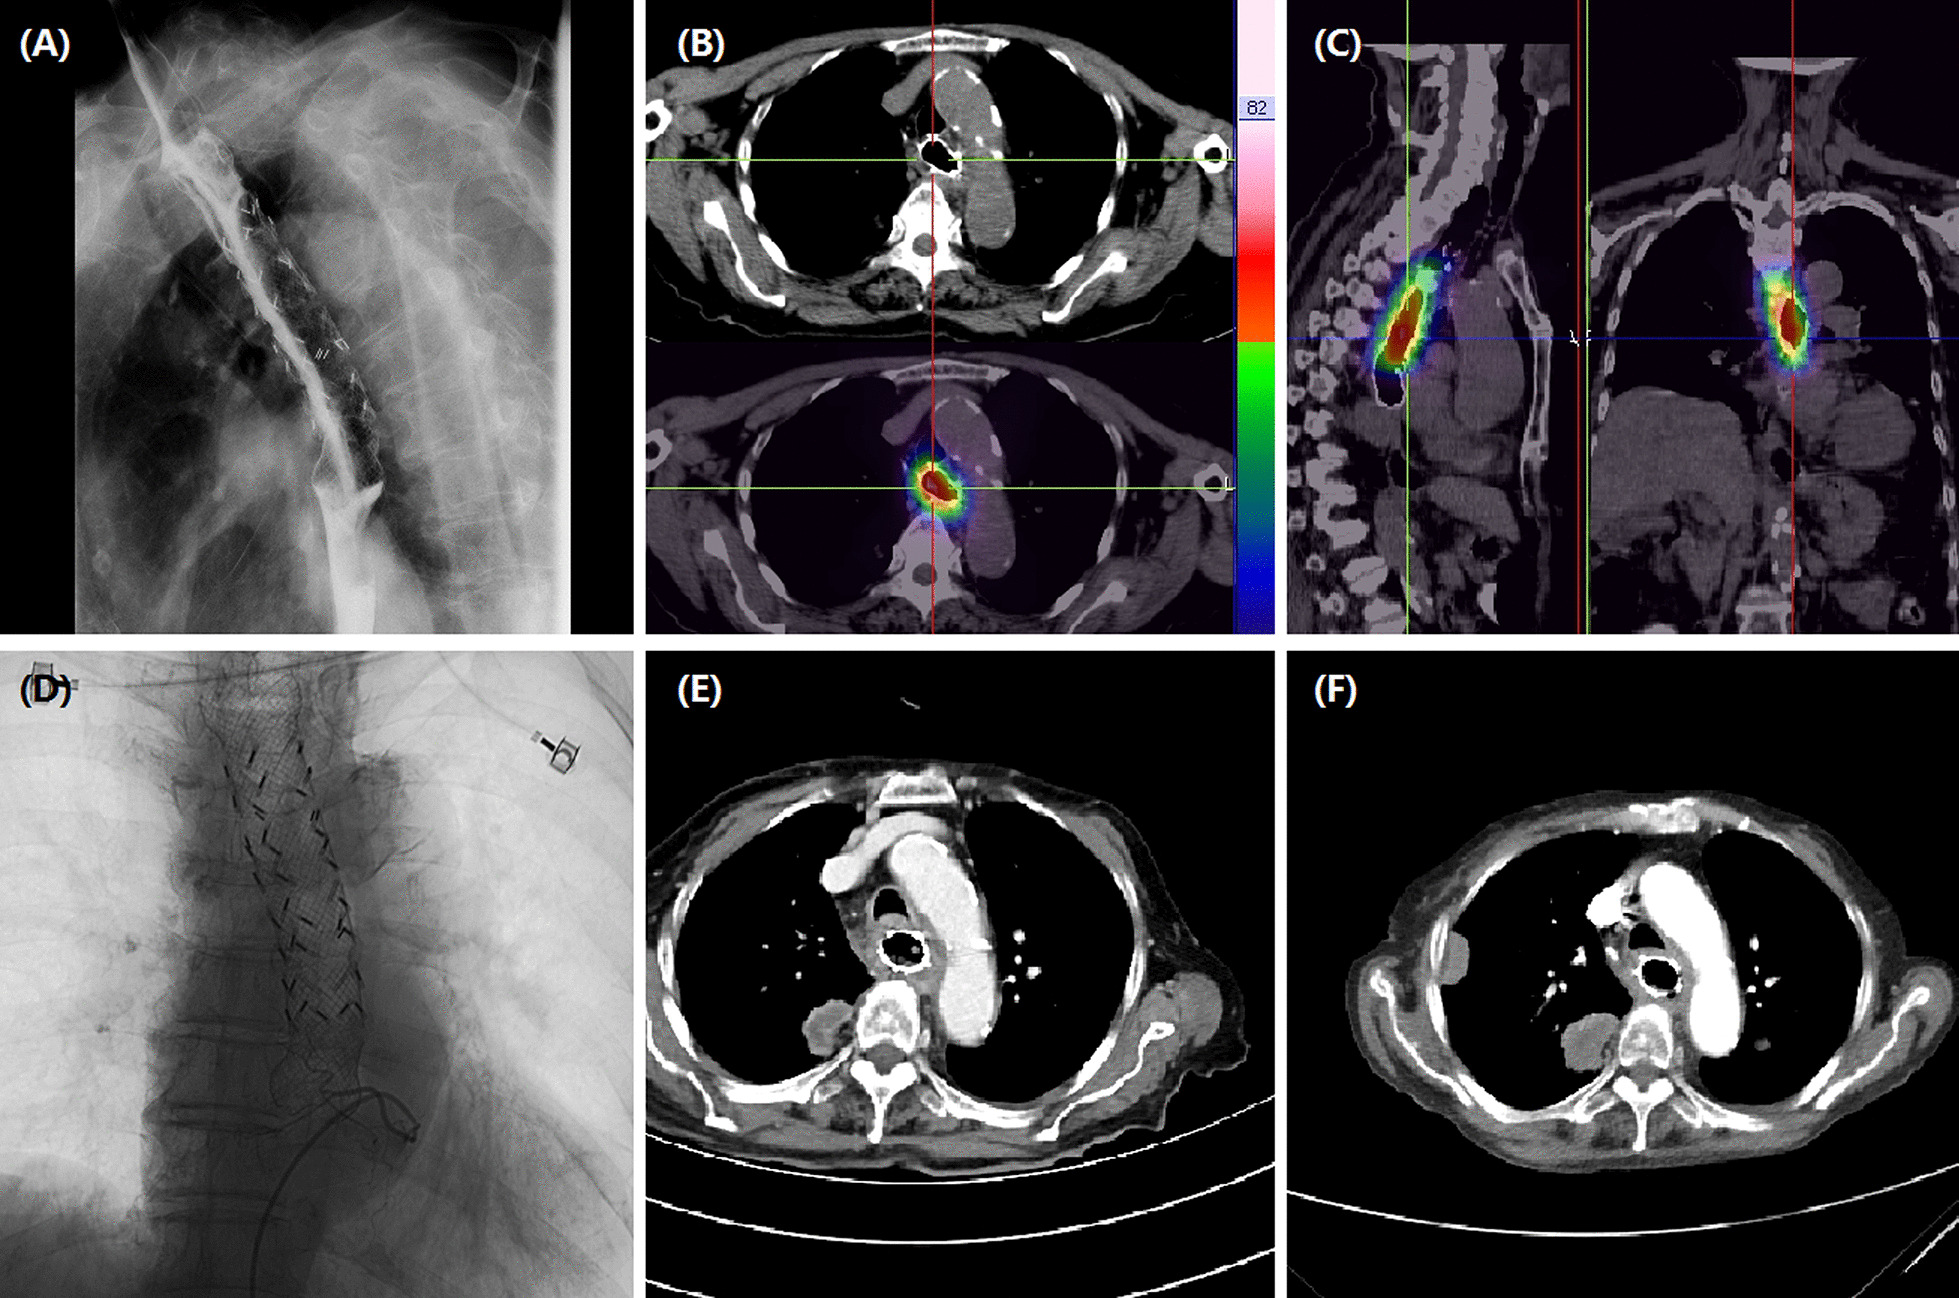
Images of an 83-year-old woman with esophageal cancer in upper and middle esophagus. aRadioactive stent was place due to serious stenosis caused by esophageal cancer. bPathological diagnosis of adenocarcinoma. cCT scan shows an obvious thickened esophageal wall with a mass in the right upper lung after 6 months. dThe left gastric artery is super-selectively catheterized and embolized using oxaliplatin loading-CalliSpheres beads. eAfter 9.9 months, CT scan shows a decreased esophageal wall with progressed masses in the right upper lung
